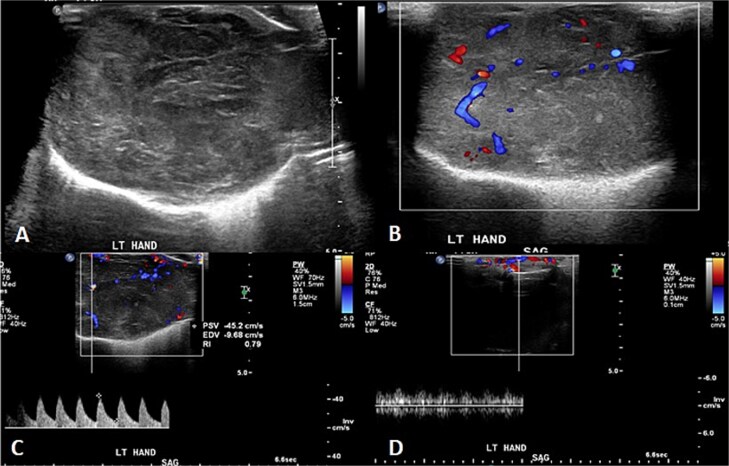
Initial imaging utilizing gray scale and color Doppler US. (A) A gray scale US image showing a large heterogeneous lesion predominantly hyperechogenic in echotexture with a partially defined margin and (B–D) color Doppler US and spectral waveform showing internal vascularity within the lesion with the presence of arterial and venous waveform. The aforementioned findings are atypical for hemangiomas.
